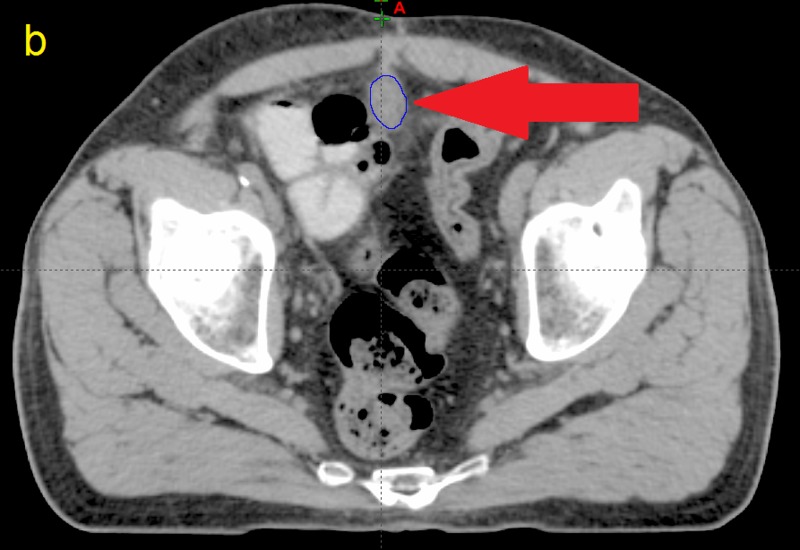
Computed tomographic (CT) image taken from patient P12 b) The same slice after contouring (blue)
